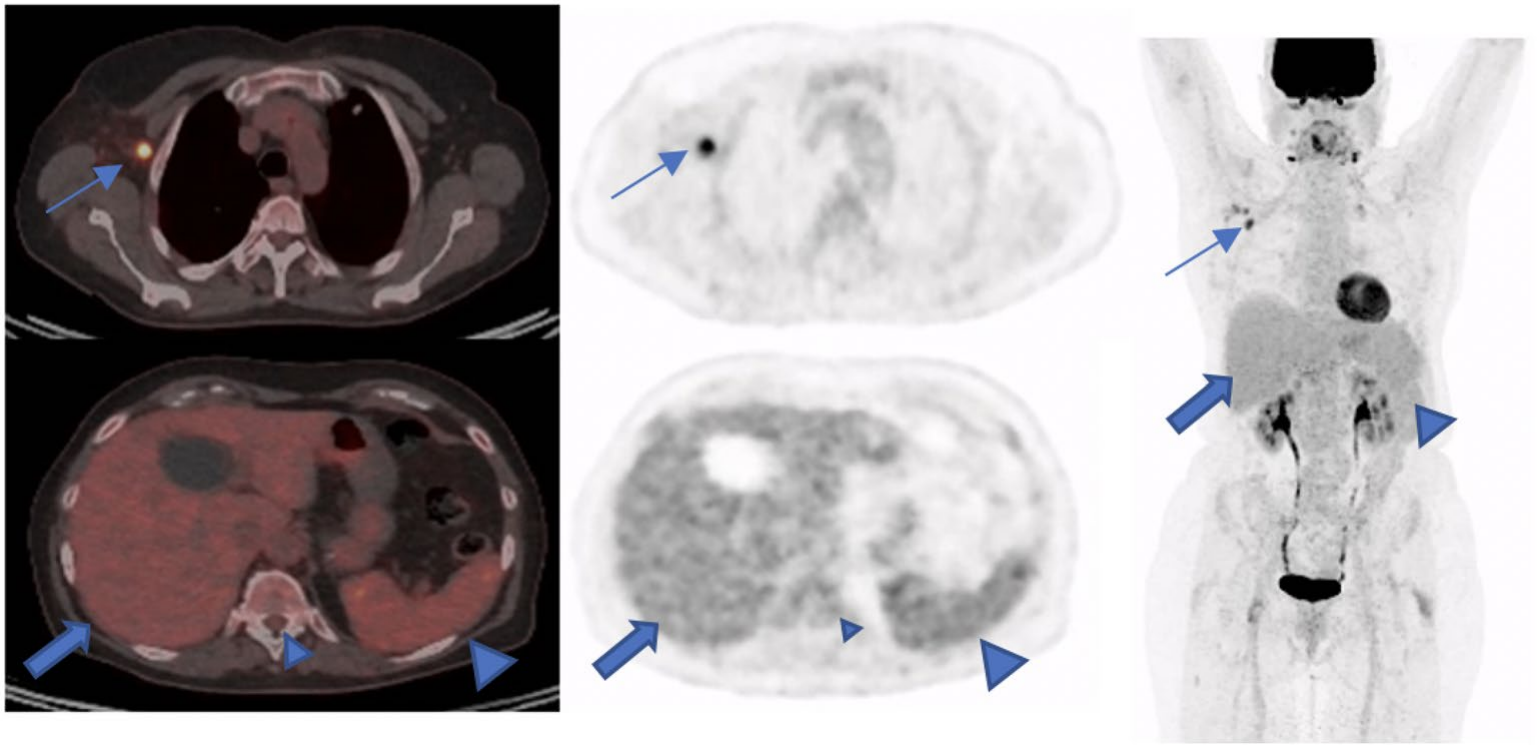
A 73-old woman with a suspicious lung nodule was referred to our division for F18-FDG PET/CT examination. She was vaccinated 4 days before PET/CT. The lung nodule did not show any pathological FDG-Uptake; however, high tracer activity was detected on her right arm, where she was injected, and multiple hypermetabolic lymph nodes in the right axilla (arrows). Moreover, PET/CT showed high tracer activity in the liver (black arrows), spleen (triangular arrows), and bone marrow (small triangular arrows) as the result of systemic immune response after vaccination. The tracer defect in the liver was due to the known cyst. (A) fusion scan, (B) PET, (C) maximum intensity projection.
